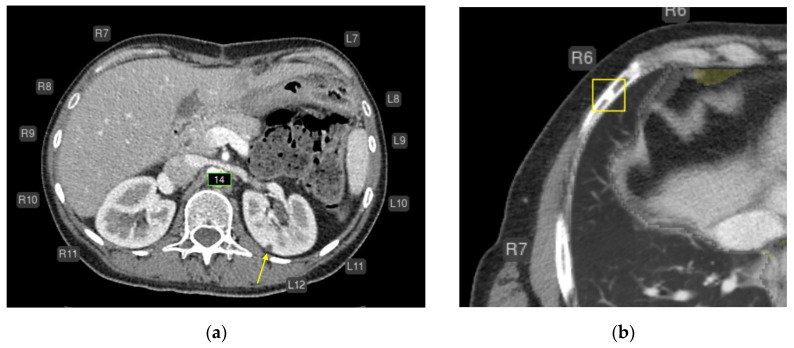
Axial CT of the abdomen (a) and chest (b) after AI processing. Missed findings without clinical significance. (a) A simple cyst in the left kidney measuring approximately 4–5 mm (yellow arrow) was not segmented (false-negative error). This is most likely a simple cyst that does not require follow-up. The AI correctly measured the abdominal aortic diameter (green) and labeled rib numbering. (b) A focal area of osteosclerosis in the anterior segment of the right 6th rib was highlighted as a consolidated fracture (yellow box; false-positive error). This finding does not require follow-up. The AI correctly labeled rib numbering and measured the aortic diameter.
